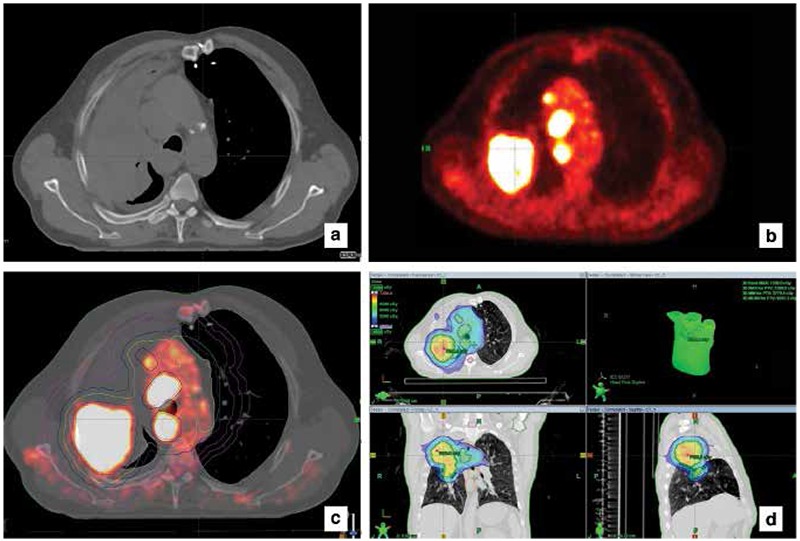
Non-small cell lung cancer, a) The atelectatic field cannot be separated from the tumor tissue in computed tomography, b) Positron emission tomography images showed increased fluorodeoxyglucose uptake in the tumor tissue, c and d) It was observed that there was a significant difference in target volumes formed by positron emission tomography/computed tomography fusion images
